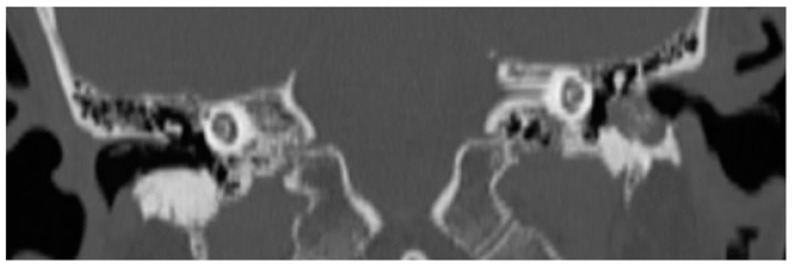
Patient 3, CT scan: evidence of soft and erosive tissue on the anterior wall of the left EAC.
